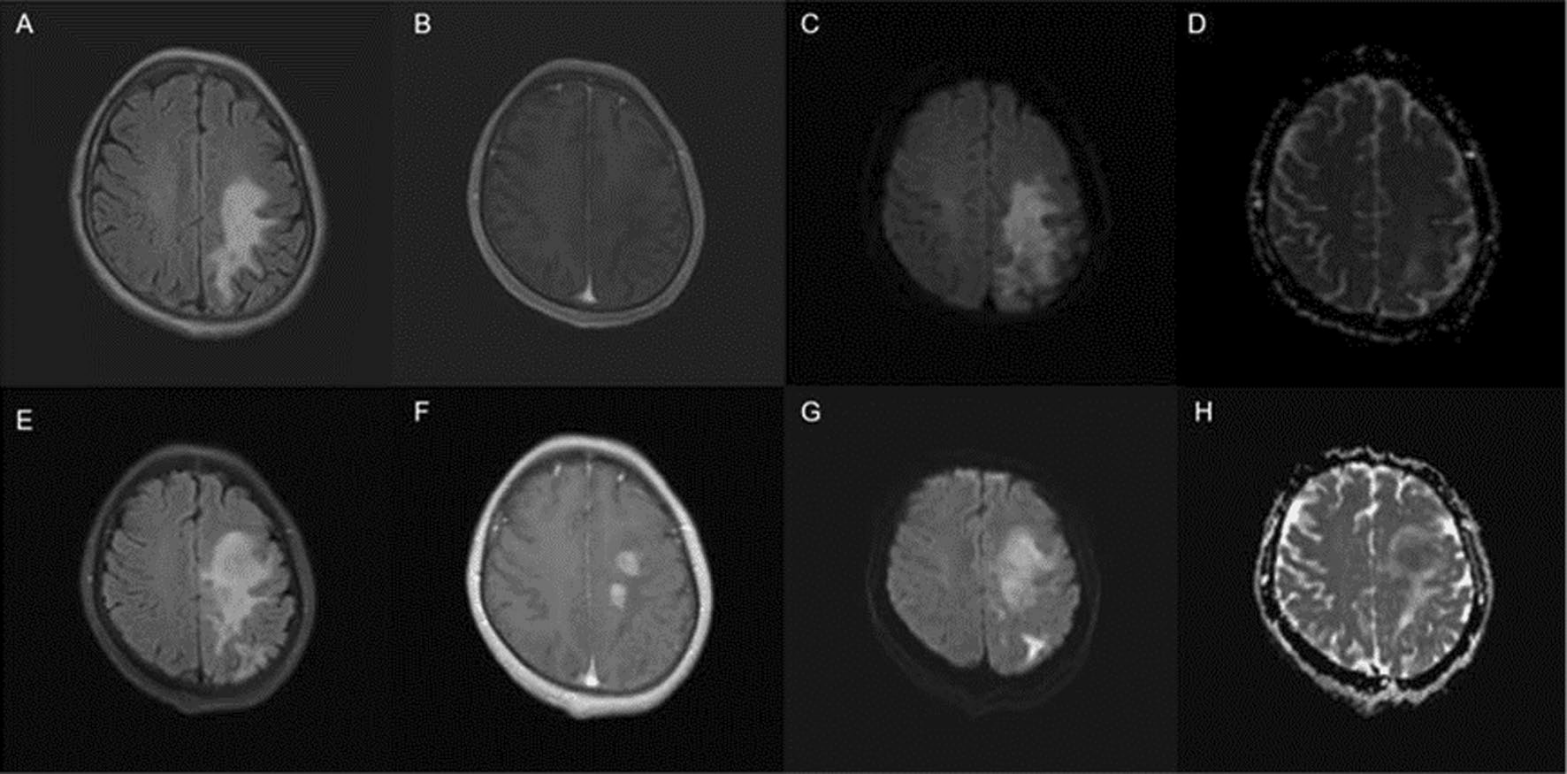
Brain MRI at initial diagnosis (A, B, C and D) and disease progression 6 months later (E, F, G and H) in a 56-year-old female patient. T2FLAIR (A) showed hyperintensity in the left frontoparietal lobe, with patchy mild enhancement on the T1 enhanced sequence (B). DWI (C) and ADC (D) sequences showed hyperintensity lesion. Brain MRI of 6 month follow-up showed a significantly enlarged range of abnormal signals on T2FLAIR (E) and obvious nodular enhancement on T1 enhanced sequences (F), hyperintense on DWI (G) and heterogeneous signal (hypointense of the center and hyperintense of the circum) on ADC sequences (H)
